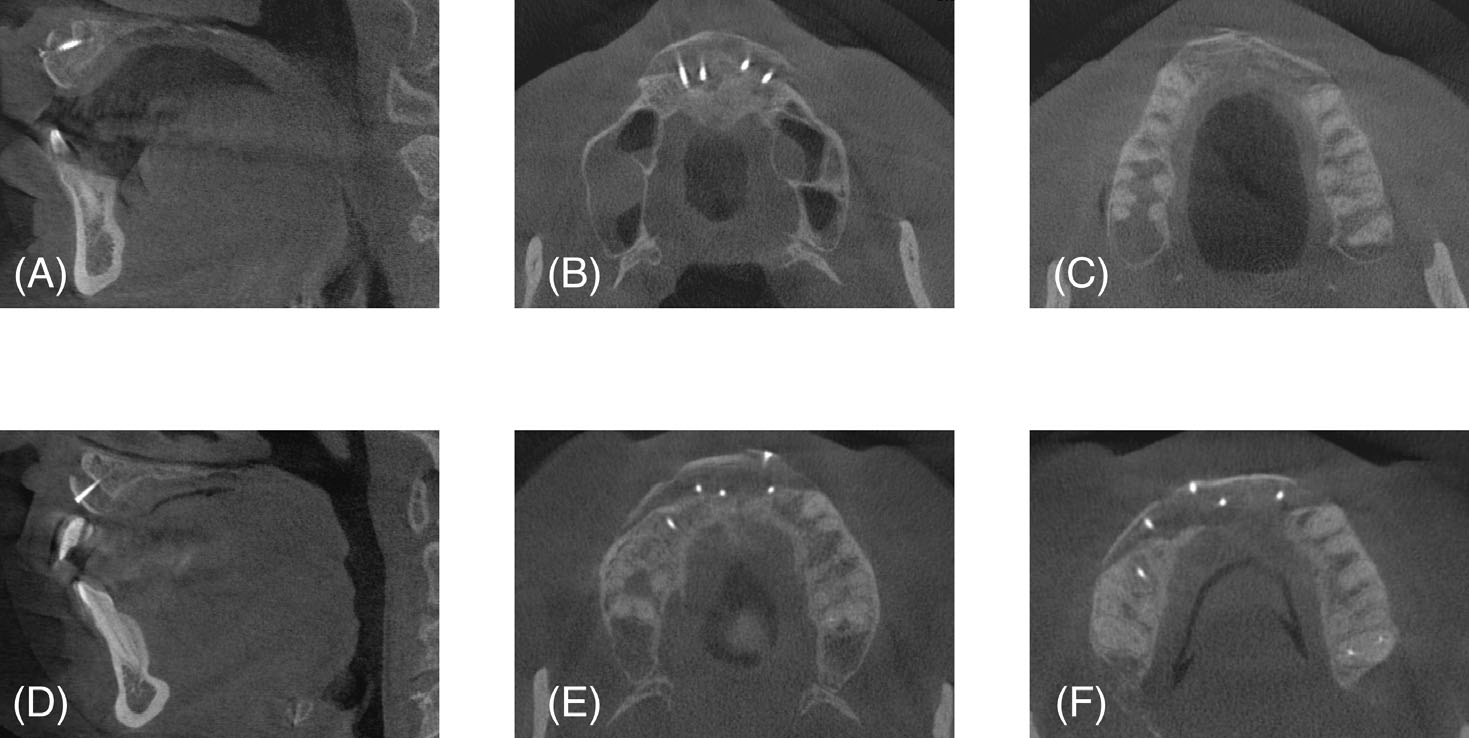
Computed tomography images of two patient cases 3 months after graft healing (A–C) patient case with reconstructed alveolar ridge in the anterior maxilla, (D–F) sagittal and axial images from a second patient case following alveolar ridge reconstruction.
